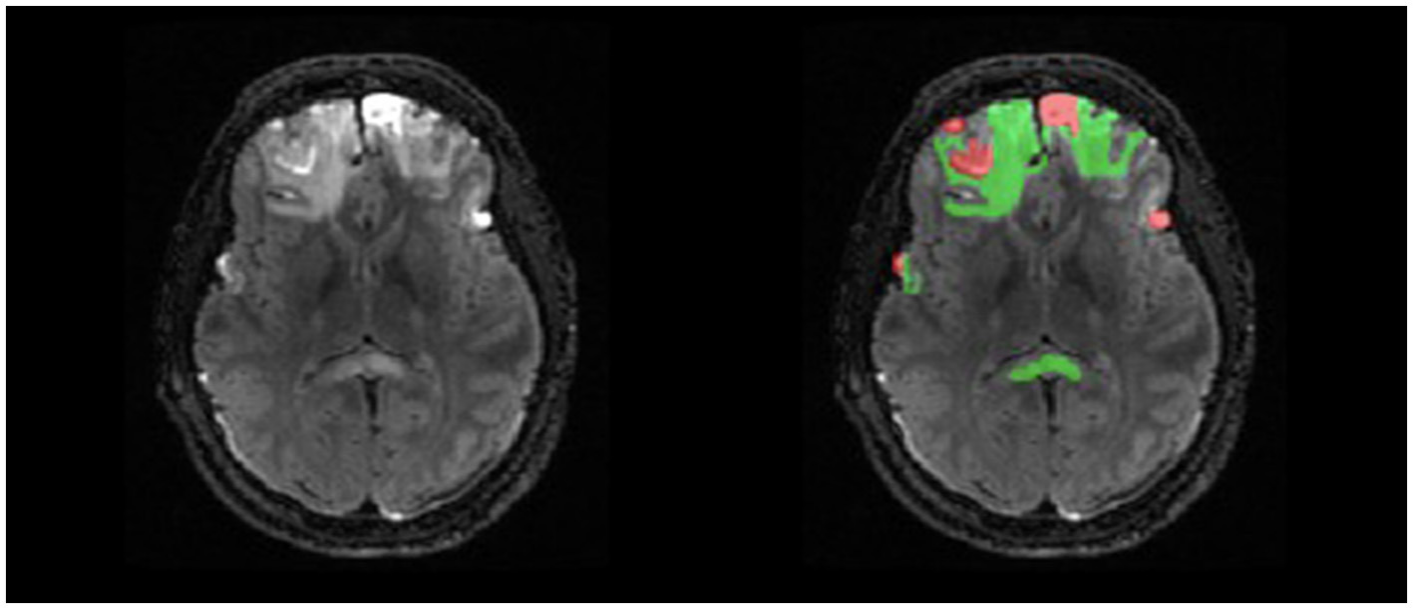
Example of double-reviewed completed parenchymal lesion segmentation on T2-FLAIR. Lesion core is depicted by the red label and surrounding edema is depicted by the green label.
